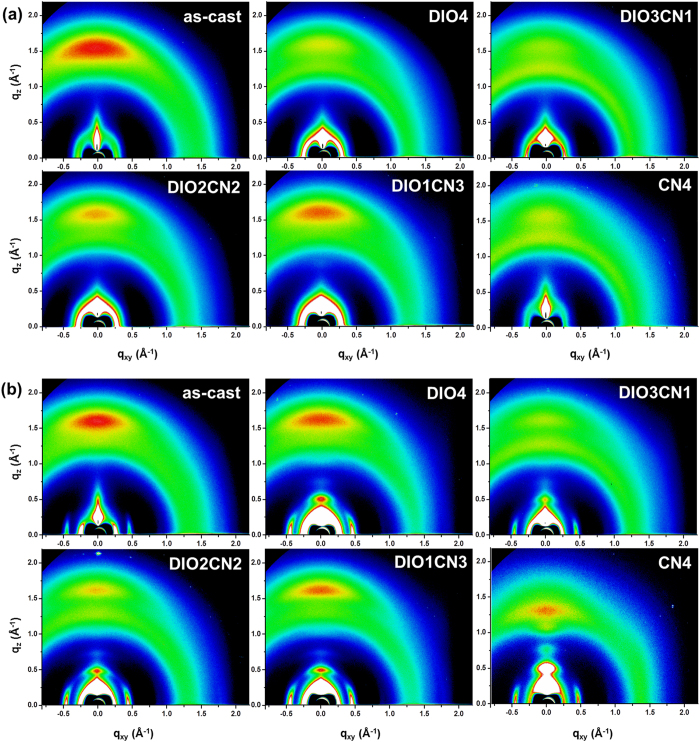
2D-GIWAXS patterns for pure PBDTTT-CT (upper line) and P(NDI2OD-T2) (lower line) films from different solutions.
